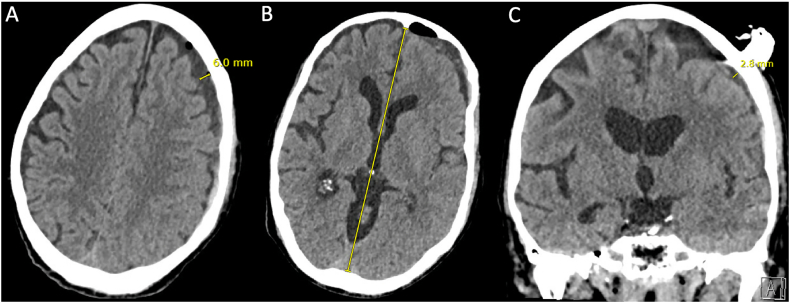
Head CT, post-procedure day 2. Caption: Head CT post-procedure day 2 after SEPS implantation. Panel A and C show further decrease in the thickness of the left convexity SDH, now 6 mm frontally and 3 mm posteriorly. Panel B shows that the prior midline shift has resolved. CT = computed tomography; SEPS = subdural evacuation port system; SDH = subdural hematoma.
